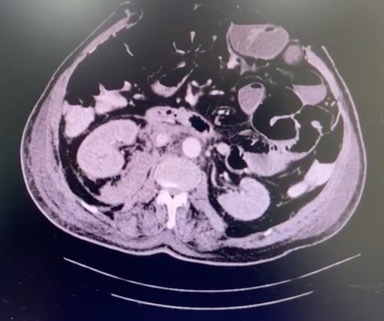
Abdominal computed tomography scan of the patient after surgical intervention, with absence of the aortocaval fistula.
